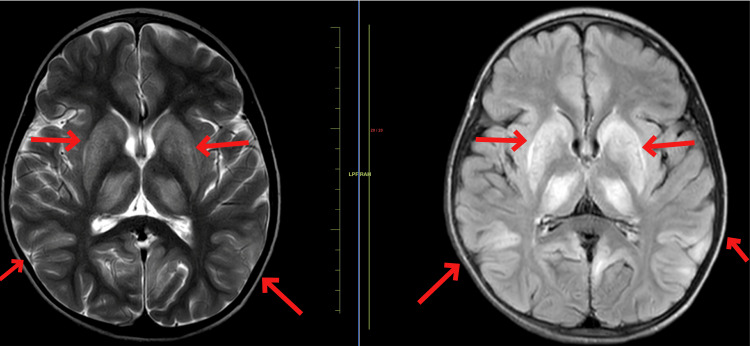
MRI showing T2/FLAIR symmetrical hyperintensities noted in the bilateral temporal lobe with sparing of occipital lobes. T2/FLAIR symmetrical hyperintensities noted in bilateral basal ganglia including corpus striatum, putamen, and thalamus.⁠ MRI: Magnetic resonance imaging; FLAIR: fluid-attenuated inversion recovery
